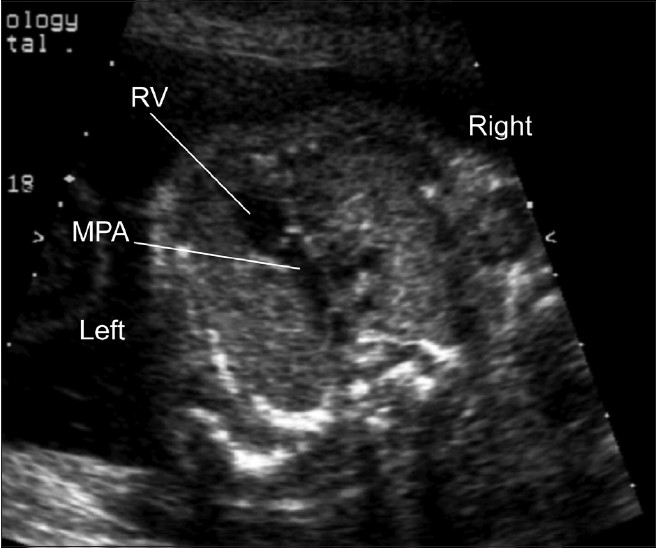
Normal pulmonary artery. The main pulmonary artery (MPA) arises anteriorly from the right ventricle (RV) and passes directly posterior toward the fetal spine. Thus, its orientation is completely different from the aorta as it leaves the heart. This “crossing” pattern of the normally related great arteries is an important feature for the sonographer to note during examination of the outflow tracts
